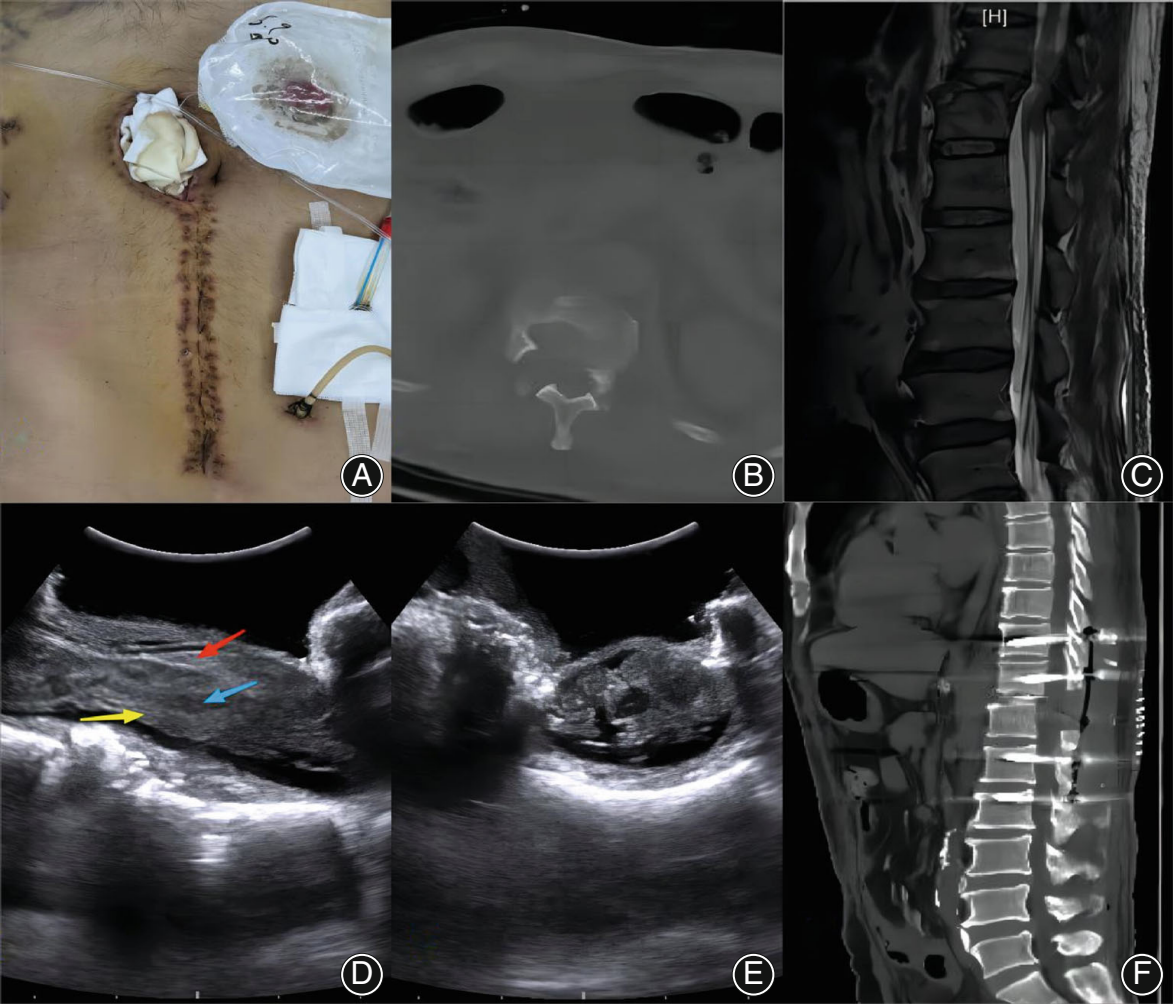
Case 1: The patient had pelvic fracture, enterostomy, and T‐tube drainage, and partial necrosis and dehiscence of the abdominal incision (A). Computed tomography (CT) and magnetic resonance imaging (MRI) showed comminuted fracture of T12 vertebral body and signal changes of spinal cord (B, C). The intraoperative ultrasound observation in lateral decubitus position showed that the spinal cord swelling and thickening were obvious (D), the injured spinal cord echo was heterogeneous (E), with slightly hyperechoic (D red arrow), isoechoic (D yellow arrow), and hypoechoic areas (D blue arrow). Postoperative CT showed satisfactory spinal canal decompression and reduction of fracture and good internal fixation position (F).
